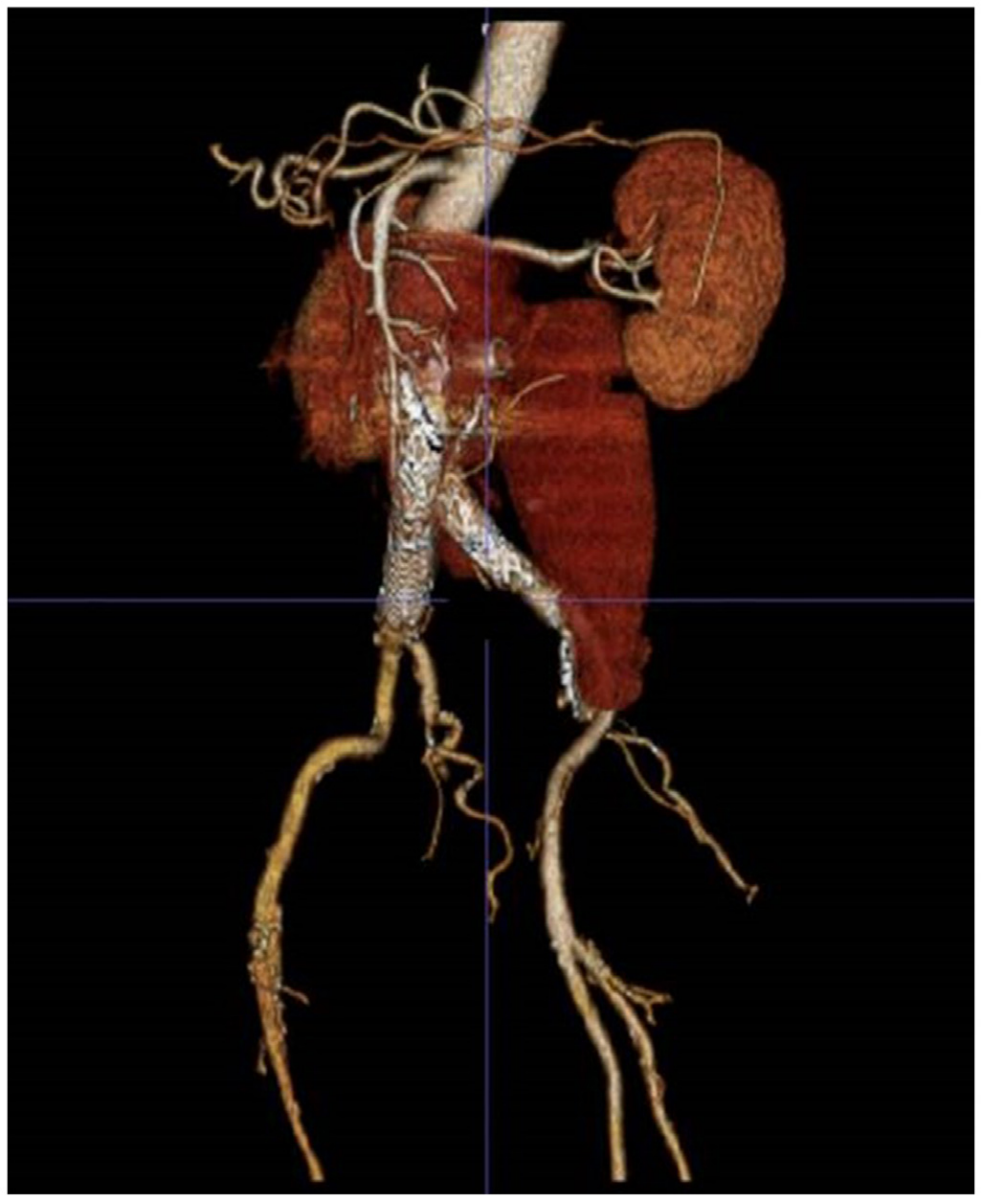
Three-dimensional reconstruction of a computed tomography scan demonstrating air and fluid adjacent to the aortic stent graft.
